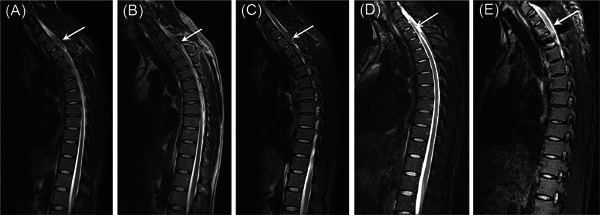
MRI image of the patient. (A, B) Day 7 of symptom onset. Both (A) and (B) show MRI images taken at the time of presentation at another hospital, showing high density of the spinal cord with suspected myelitis 7 days after the onset of the disease. (C) Day 16+ of symptom onset. MRI showed a T1–T2 fusiform mass that was isodense on the sagittal T1‐weighted image and hyperintense on the T2‐weighted image, but hyperintense on the T1‐weighted image in the center of the mass. (D) Day 22+ of symptom onset. Preoperative contrast‐enhanced MRI revealed that there was a significant enhancement, but uneven expansion of the fusiform mass to the level of the C7–T2 vertebral body in the sagittal position. (E) 12 months after surgery. MRI in thoracic vertebra showed decompression of the spinal canal at the level of the C7–T2 vertebra. MRI, magnetic resonance imaging.
